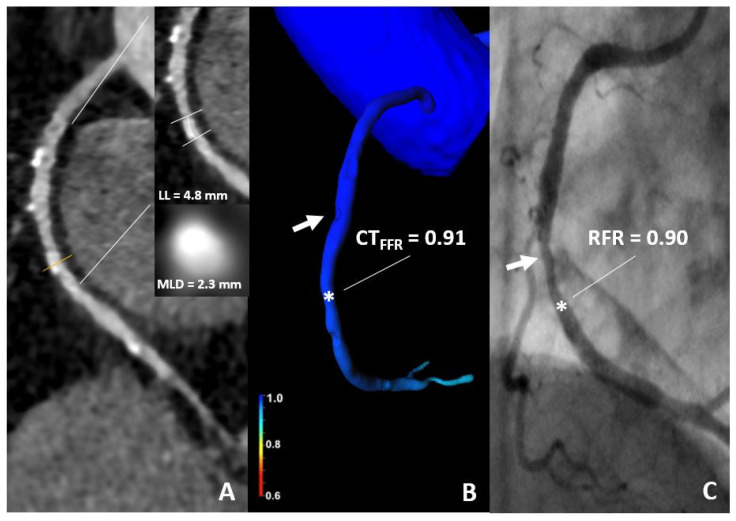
86-year-old woman. (A) CT demonstrates a 60% stenosis of the mid segment of the RCA. (B) The CT-FFR value was 0.91 (star). (C) An ICA with RFR value of 0.90 (star) shows a non-significant stenosis. CT, computed tomography; CT-FFR, coronary computed tomography angiography based fractional flow reserve; RFR, resting full cycle Ratio; LL, lesion length; MLD, minimal luminal diameter.
